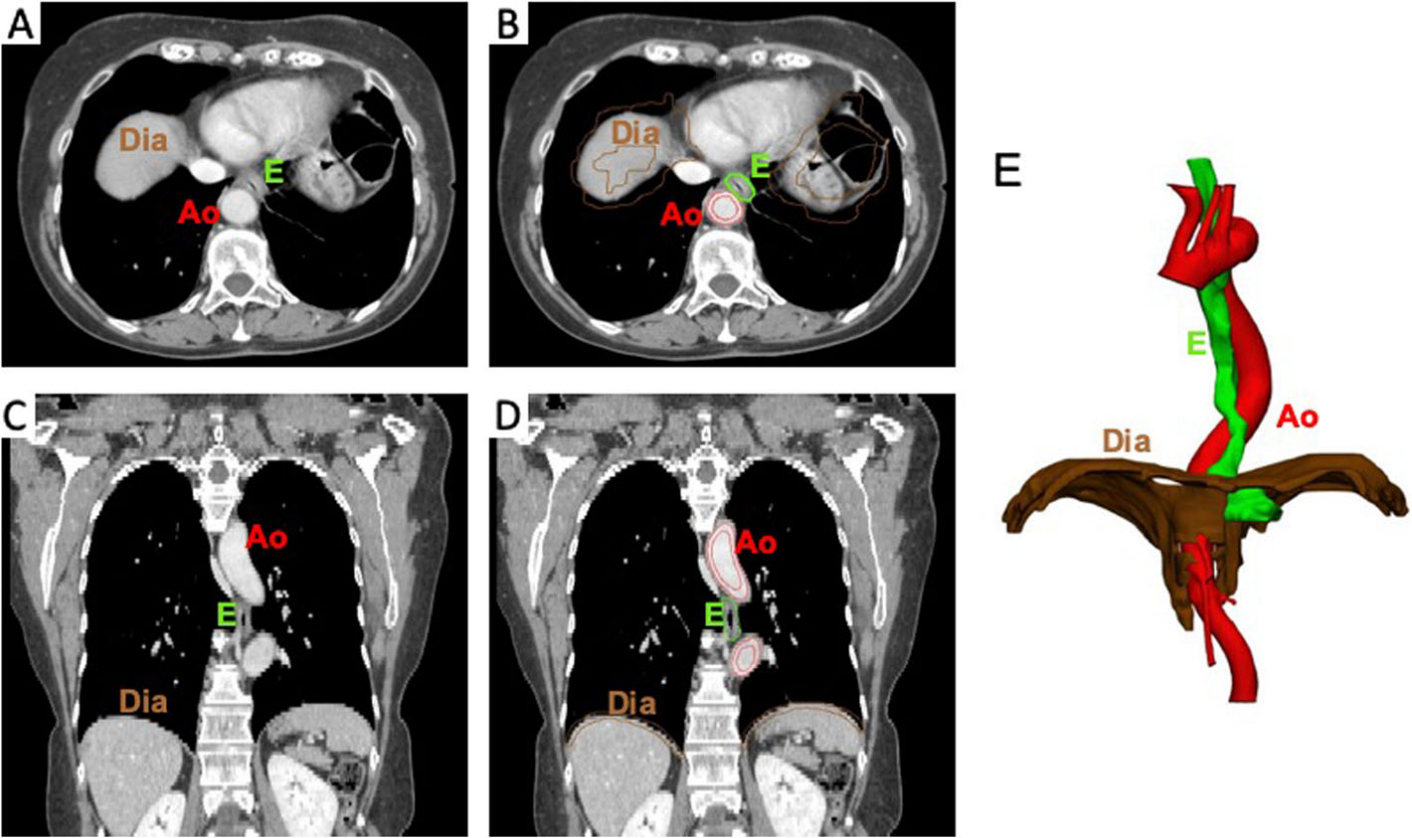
(a) Basal axial (transverse) image of CT scans of the thorax/upper abdomen. (b) Segmented axial (transverse) image of CT scans of the thorax/upper abdomen. (c) Basal coronal image of CT scans of the thorax/upper abdomen. (d) Segmented coronal image of CT scans of the thorax/upper abdomen. (e) Final 3D reconstruction. E, Esophagus; Ao, Aorta; Dia, Diaphragm
